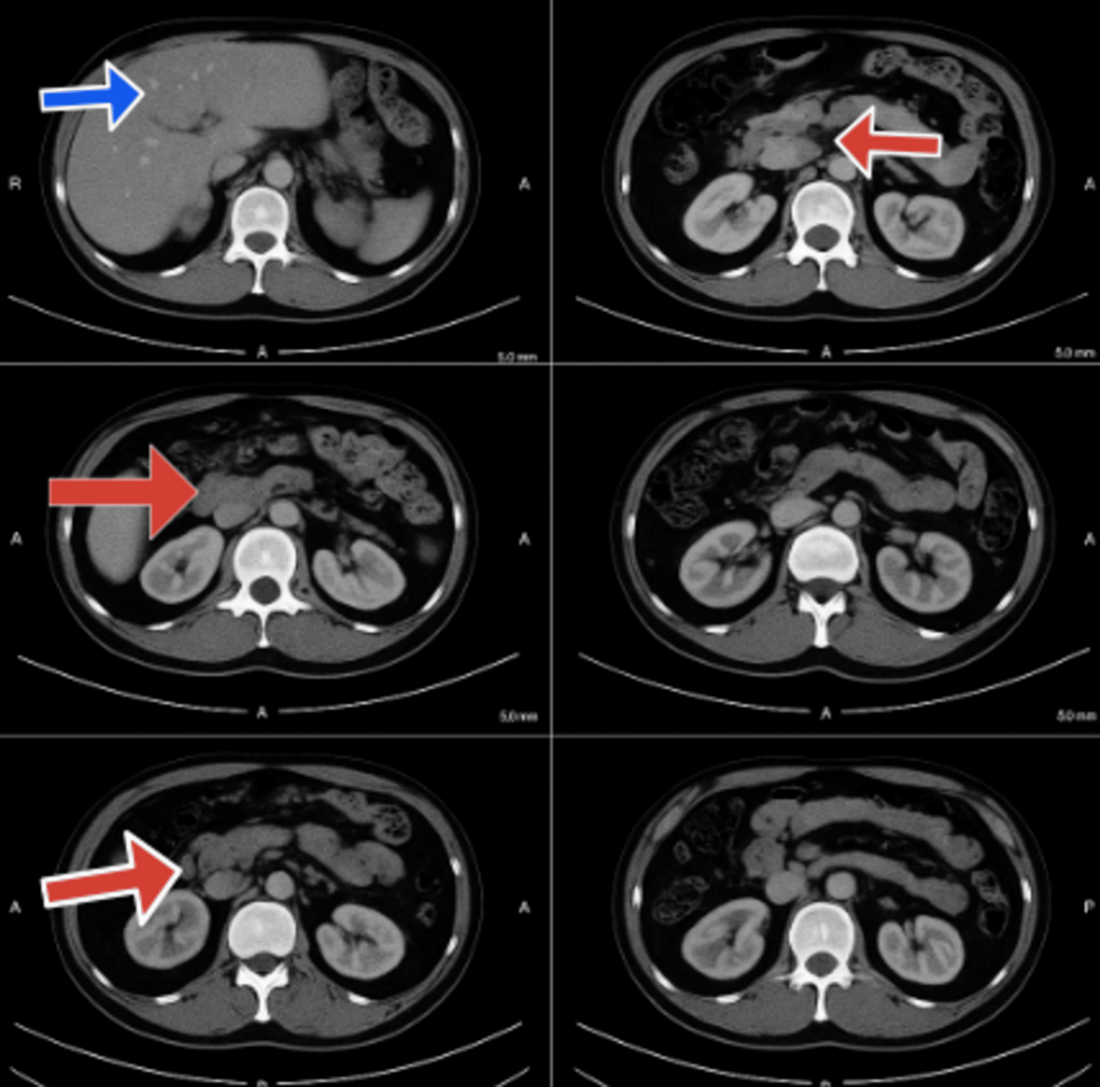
CT abdomen showing pancreatic mass with liver metastasis CT: Computerized Tomography The red arrows show the pancreatic mass, while the blue arrow shows the hepatic metastatic lesions.
